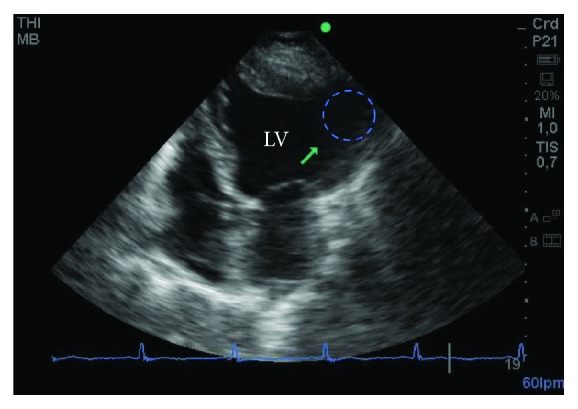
The echocardiogram reveals a large aneurysm (diameter, 3 cm; encircled area) in the lateral basal-medial region of the ventricle without apparent thrombus (arrow). LV: left ventricle.
